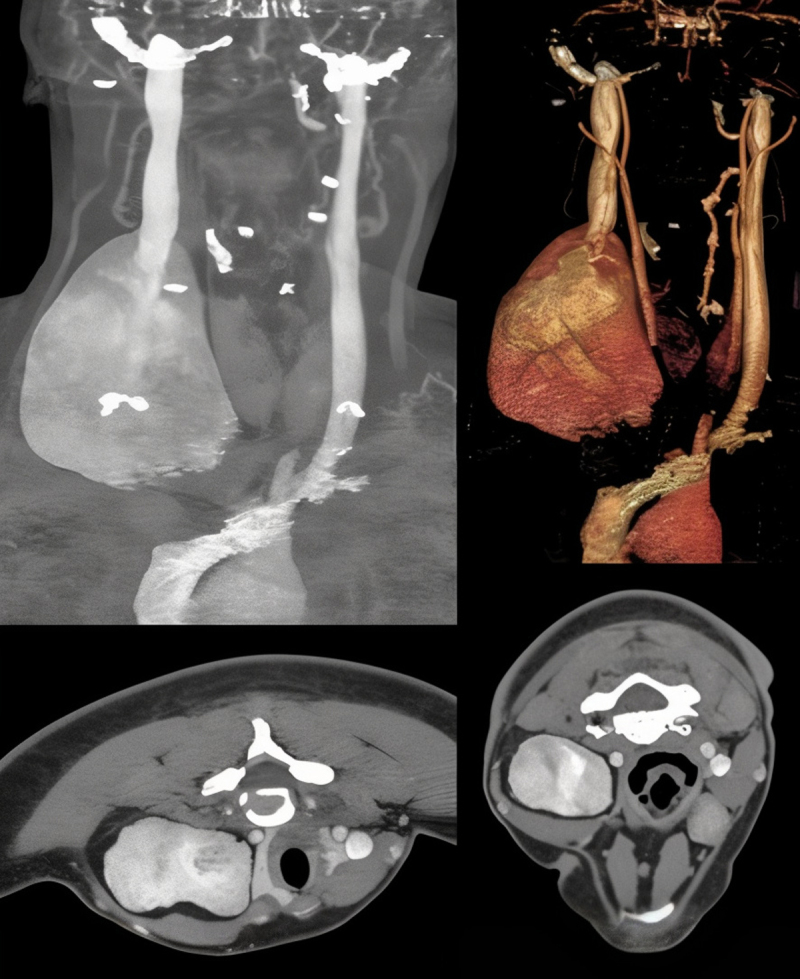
Preoperative computed tomography cerebral venography imaging of the internal jugular vein aneurysm.
